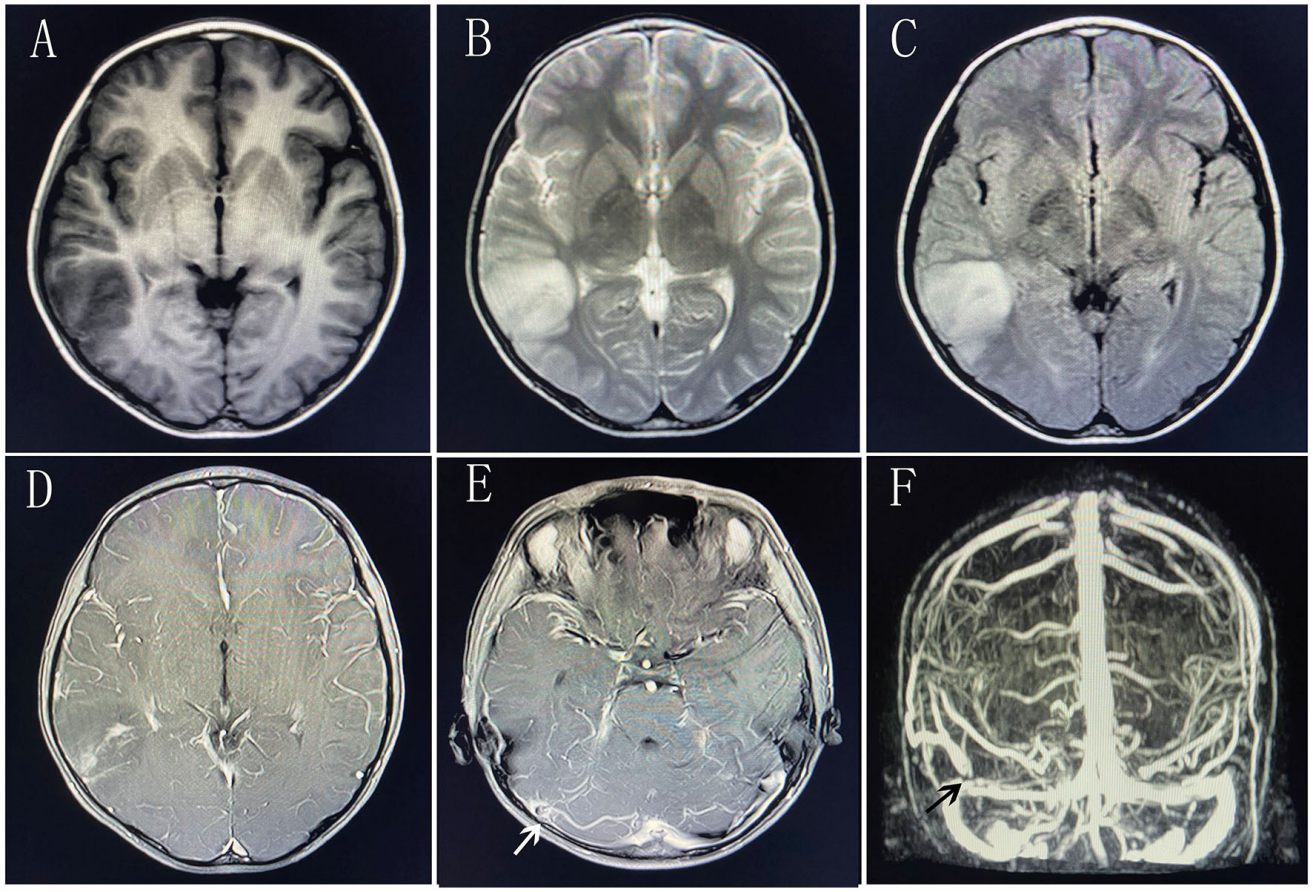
Brain magnetic resonance imaging at first administration. (A) T1-weighted image. (B) T2-weighted image. (C) Flair sequence. (D) Gadolinium-enhanced T1-weighted image of the right temporal lobe. (E) Gadolinium-enhanced T1-weighted image of the transverse sinus. The white arrow indicates the filling defect of right transverse sinus. (F) MRV scanning. The black arrow indicates the interruption of blood flow at the junction of the right Labbe vein and right transverse sinus.
